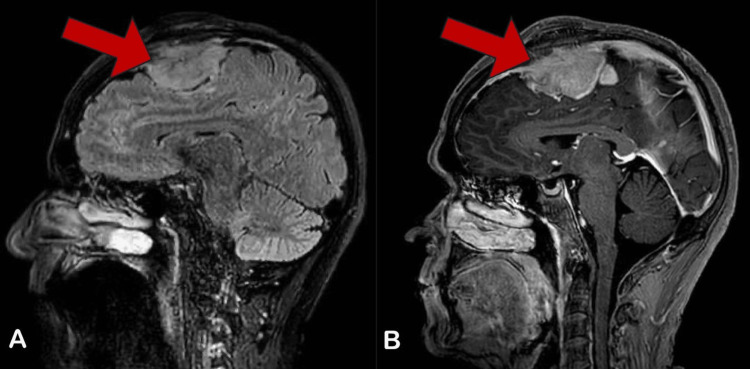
(A) Sagittal T1-Weighted MRI and (B) Sagittal T2-Weighted MRI Demonstrate a Large Anterior Superior Extra-axial Mass With a Lobulated Margin. Mass Effect on Corpus Callosum and Anterior Bodies of Lateral Ventricles Was Noted.
